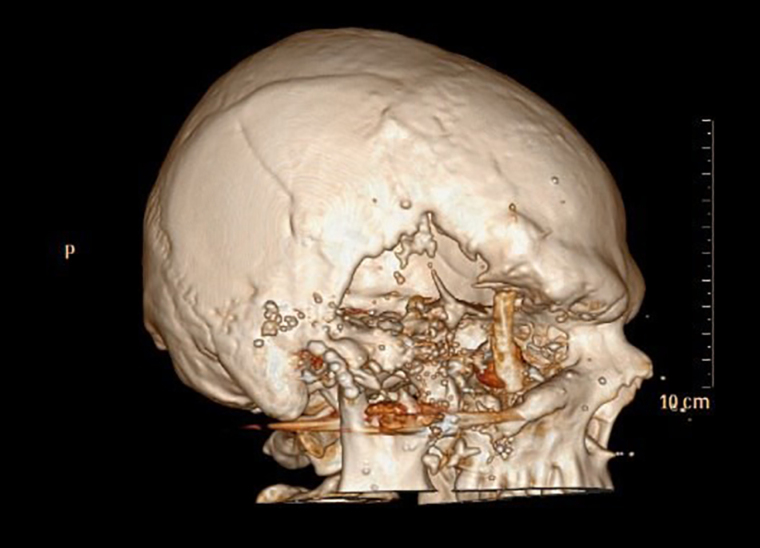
Postoperative 3D CT reconstruction (lateral view) clearly showing the iliac crest graft securely integrated at the reconstructed lateral orbital rim. 3D = three-dimensional, CT = computed tomography.
